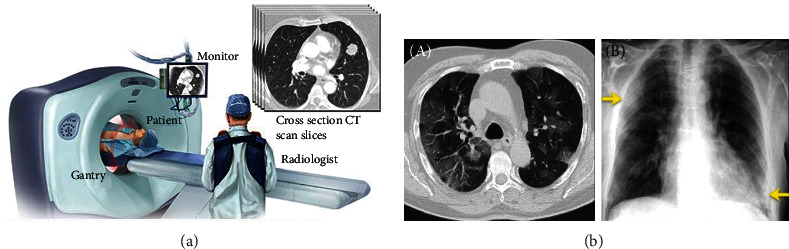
(a) Radiological image acquisition (courtesy: newsnetwork.mayoclinic.org/discussion/mayo-clinic-radio-lung-cancer-updatehousehold-health-hazardsprediabetes). (b) (A) Axial chest CT image (non-enhanced) of a positive RT-PCR-confirmed 70-year-old man showing ground-glass opacities along with dilated segmental and subsegmental vessels prominent on the right side. (b) (B) CXR showing pulmonary hypertension, mitral insufficiency, and atrial fibrillation along with COVID-19 contagion in an 83-year-old man (arrows indicating ground-glass opacity findings in the upper right lobe and consolidation findings in the lower left lobe of the lungs) (arrows) (courtesy: radiologyassistant.nl).
